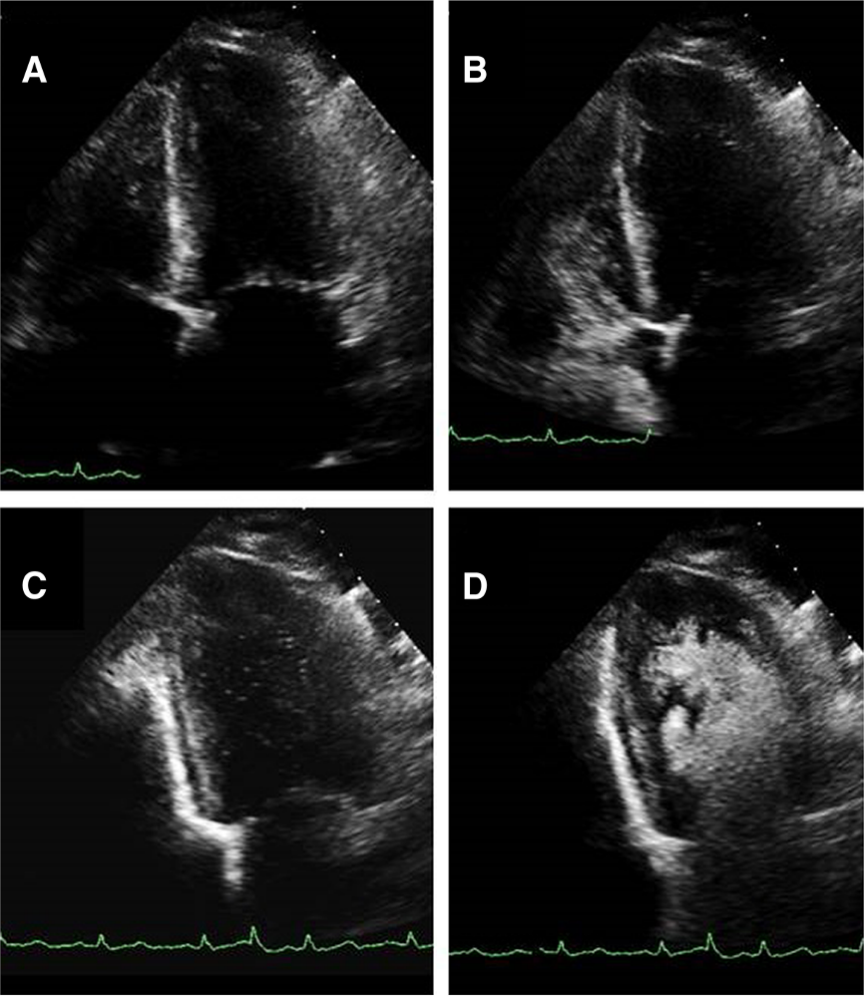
Representative apical 4-chamber view images demonstrating (A) left ventricle and right ventricle prior to contrast injection, (B) full opacification by Definity contrast of the right ventricle, (C) first appearance of contrast in the left ventricle, and (D) full opacification of the left ventricle.
